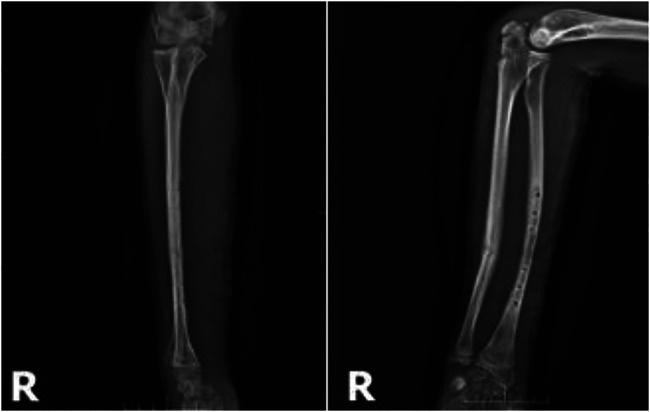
X-rays after removal of internal fixation at 5 months postoperatively.
